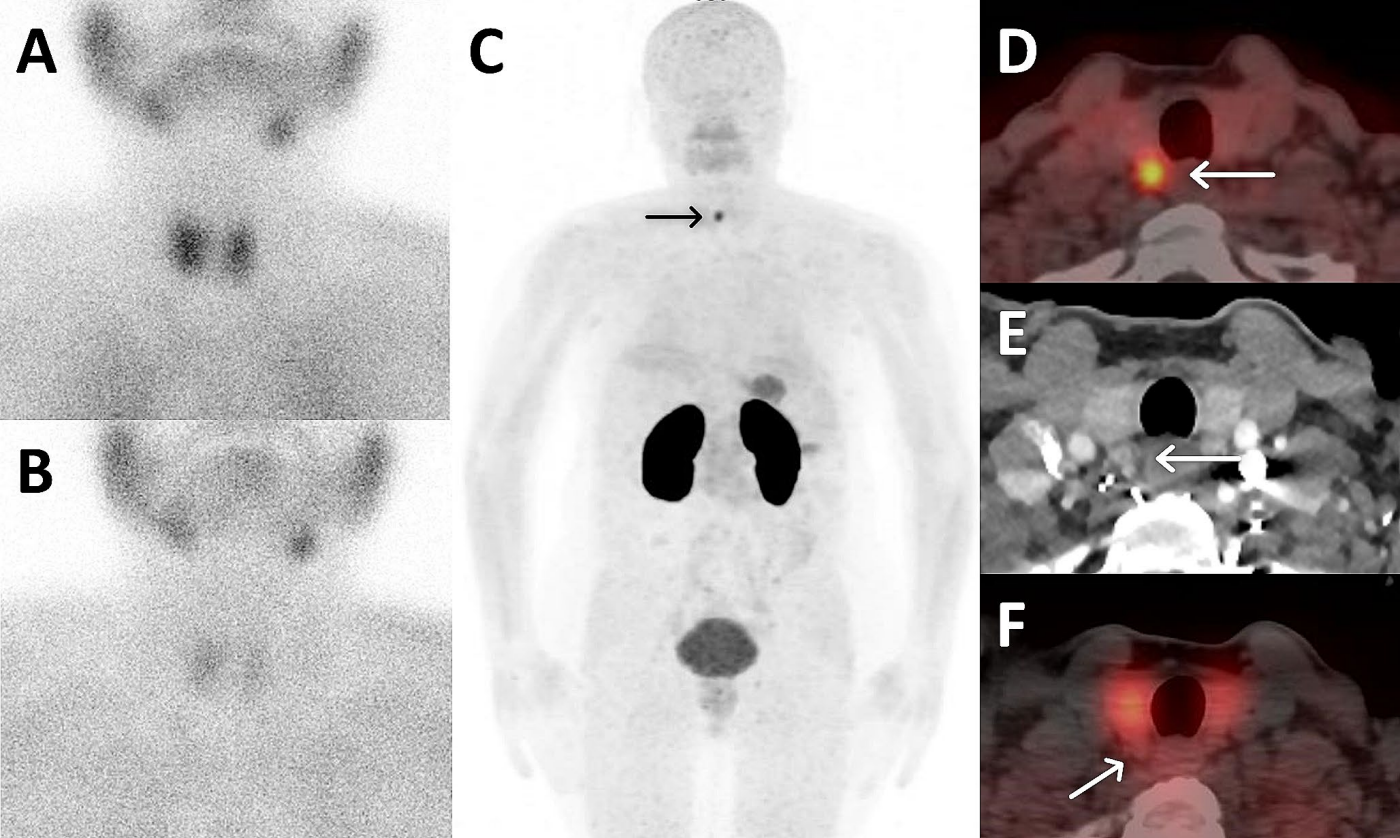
A 61-year-old woman diagnosed with PHPT (serum PTH: 118 ng/l Calcium: 10.3 mg/dL) underwent dual-phase MIBI scan showing no uptake (A, B) which prompted further investigation. Subsequent ^68^Ga-Trivehexin PET/CT displayed focal tracer activity, notably visible on maximum intensity projection images (C), located posterior to the left thyroid lobe on the axial plane (D). The lesion showing contrast enhancement (E) on 4D-CT exhibited no uptake on SPECT/CT images (F)
